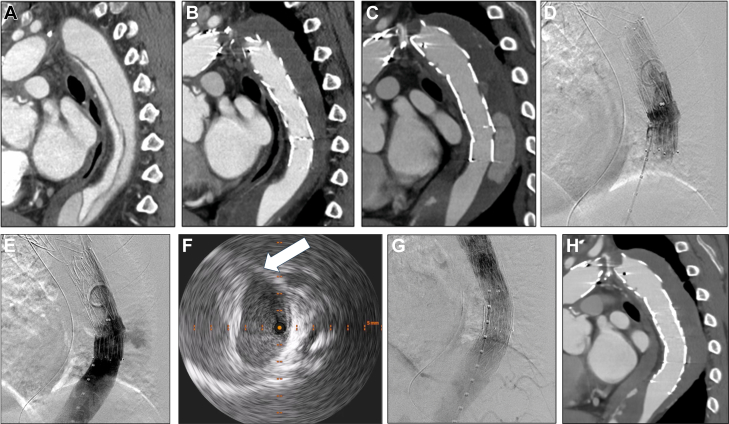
Preprocedure and Postprocedure Computed Tomography Angiography, Intraoperative Angiography, and Intravascular Ultrasound (A) No re-entry at the descending aorta after total arch replacement. (B) No endoleaks 5 years ago. (C) Endoleak at the time of visiting the hospital. (D) No type 3 endoleak. (E) No distal stent graft–induced new entry. (F) Defect of aortic wall (arrow). (G) No endoleak. (H) No endoleak.
